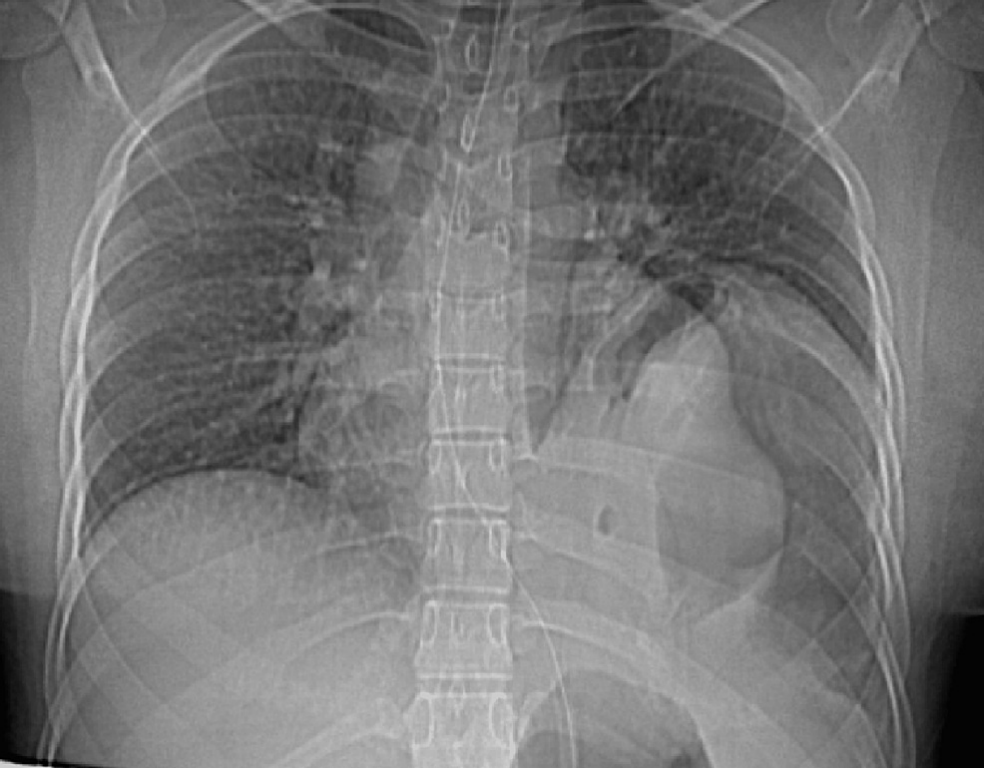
Chest radiograph AP view at presentation, after the passage of the nasogastric tube, showing the elevated left hemidiaphragm and gastric shadow. AP, anteroposterior
